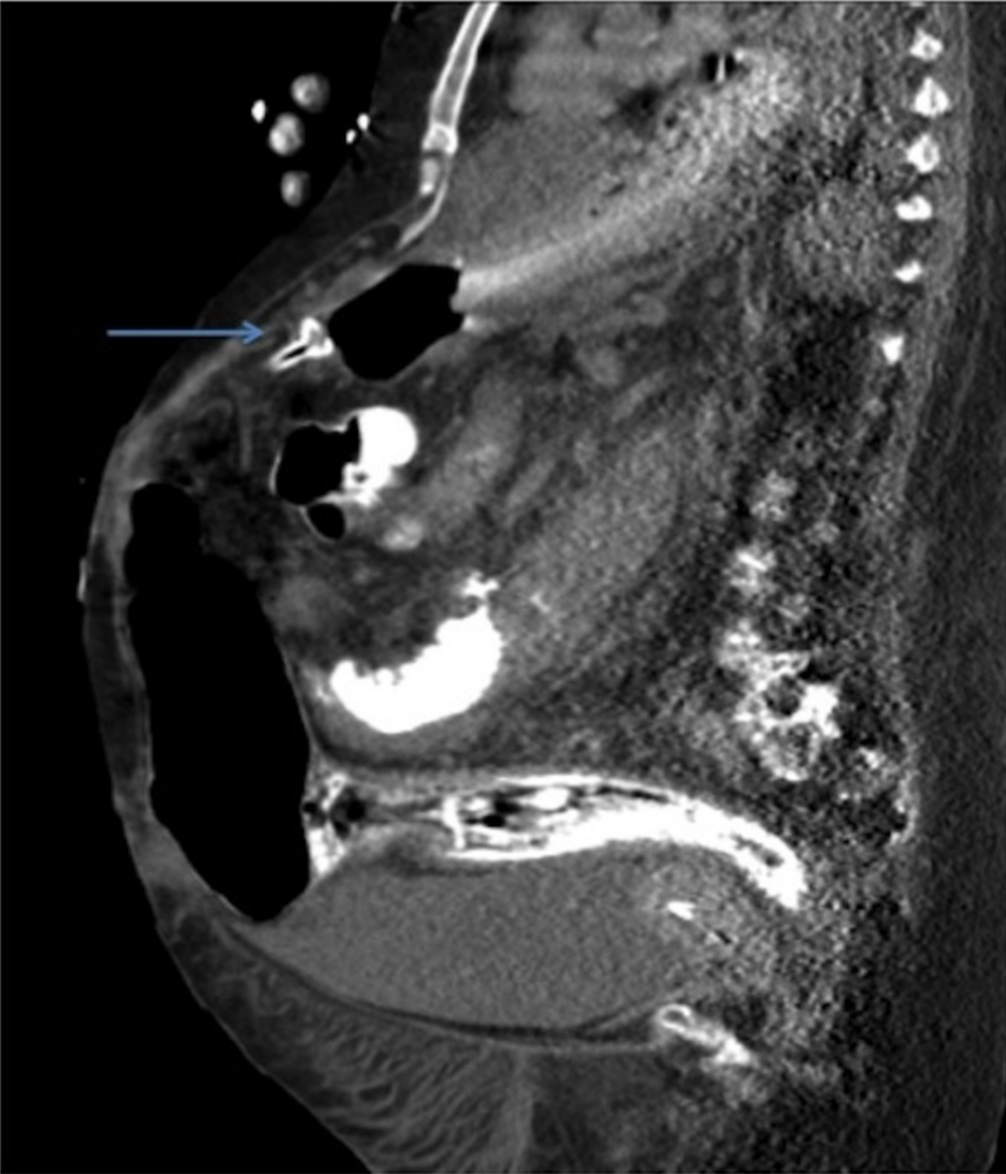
The erosion of the gastrostomy tube through the stomach wall depicted on coronal view of a computed tomography (CT) scan of the abdomen
